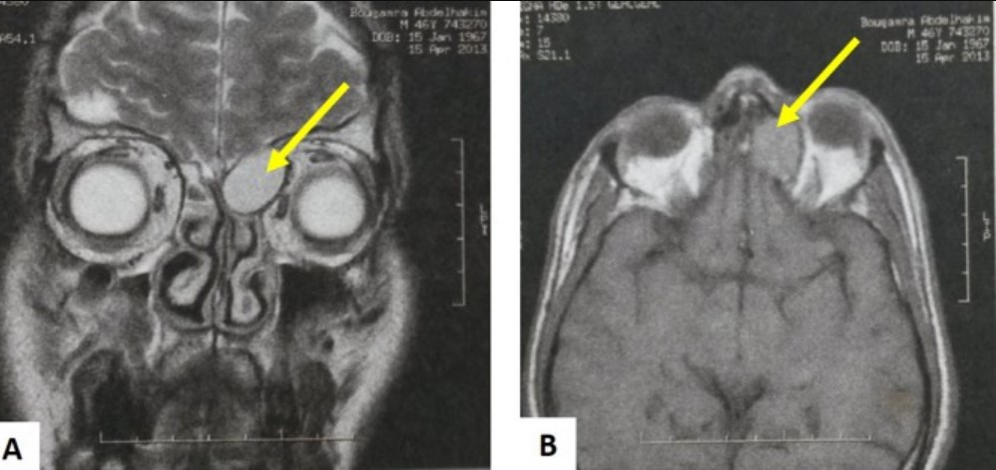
MRI of the facial area: in T1 axial section (A) and T2 frontal section (B): showing a well-defined left ethmoid cystic formation in discrete hyper-signal T1, hyper-T2 signal with intra orbital extension
